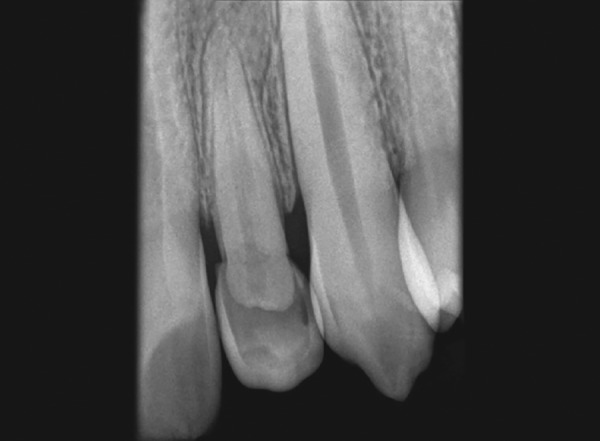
Periapical radiograph of the tooth after 18 months follow-up showing no signs of pathology
